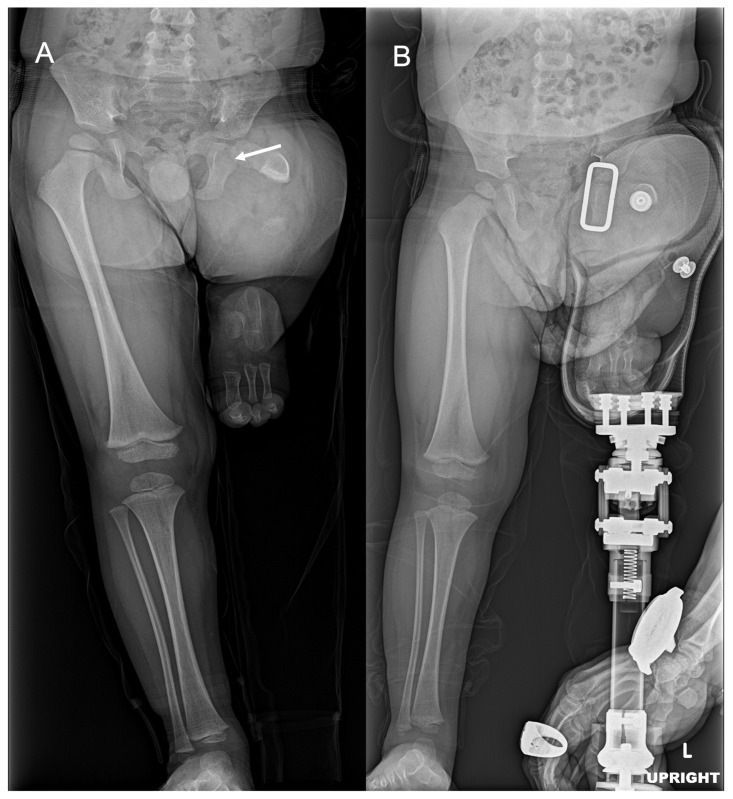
AP bilateral lower extremity radiographs with and without prosthesis. (A) Short and ossified portion of the left femoral neck and proximal diaphysis (white arrow). (B) Standing with left transfemoral prosthetic.
